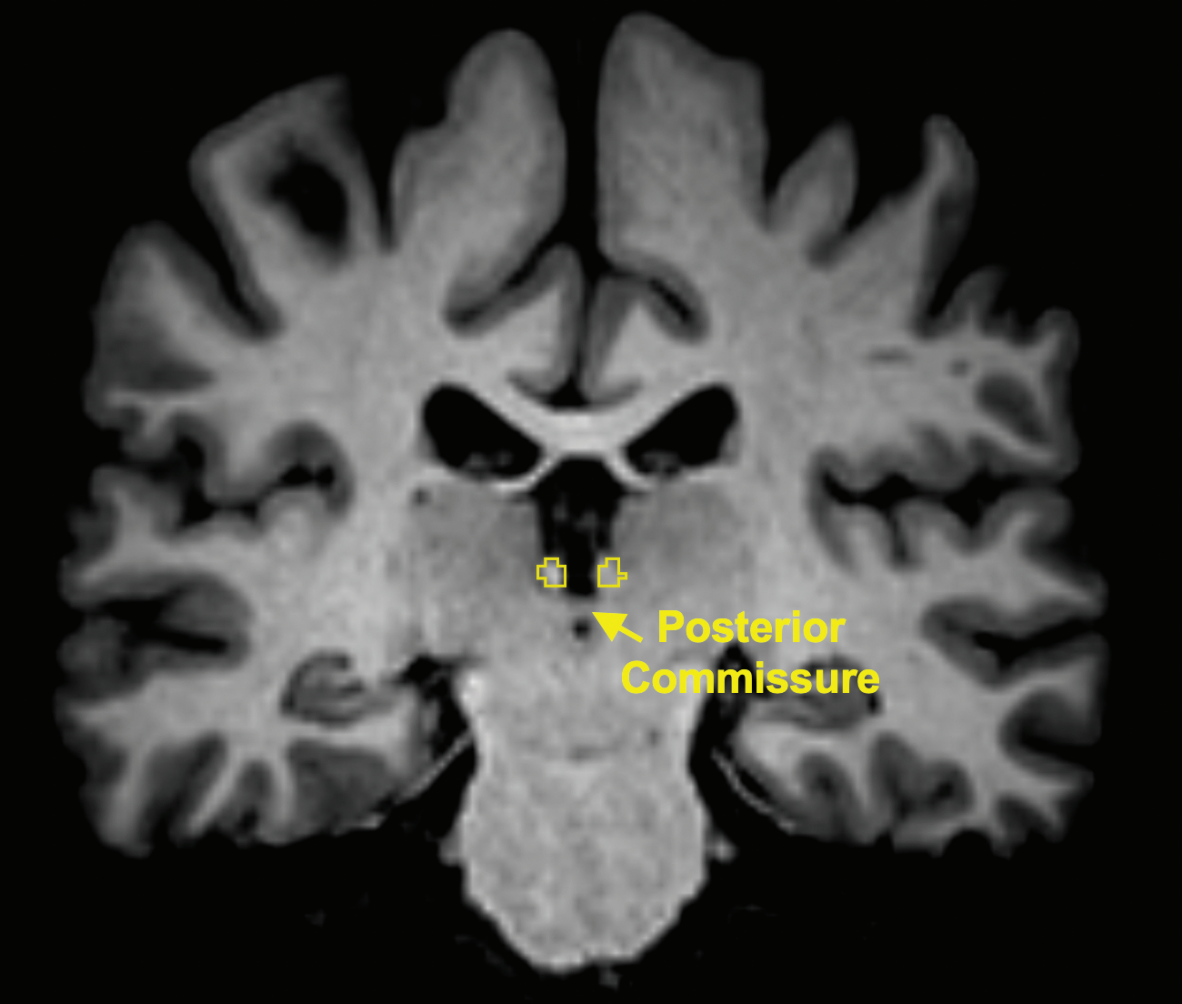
Delineation of the habenula on a T1-weighted coronal image.
